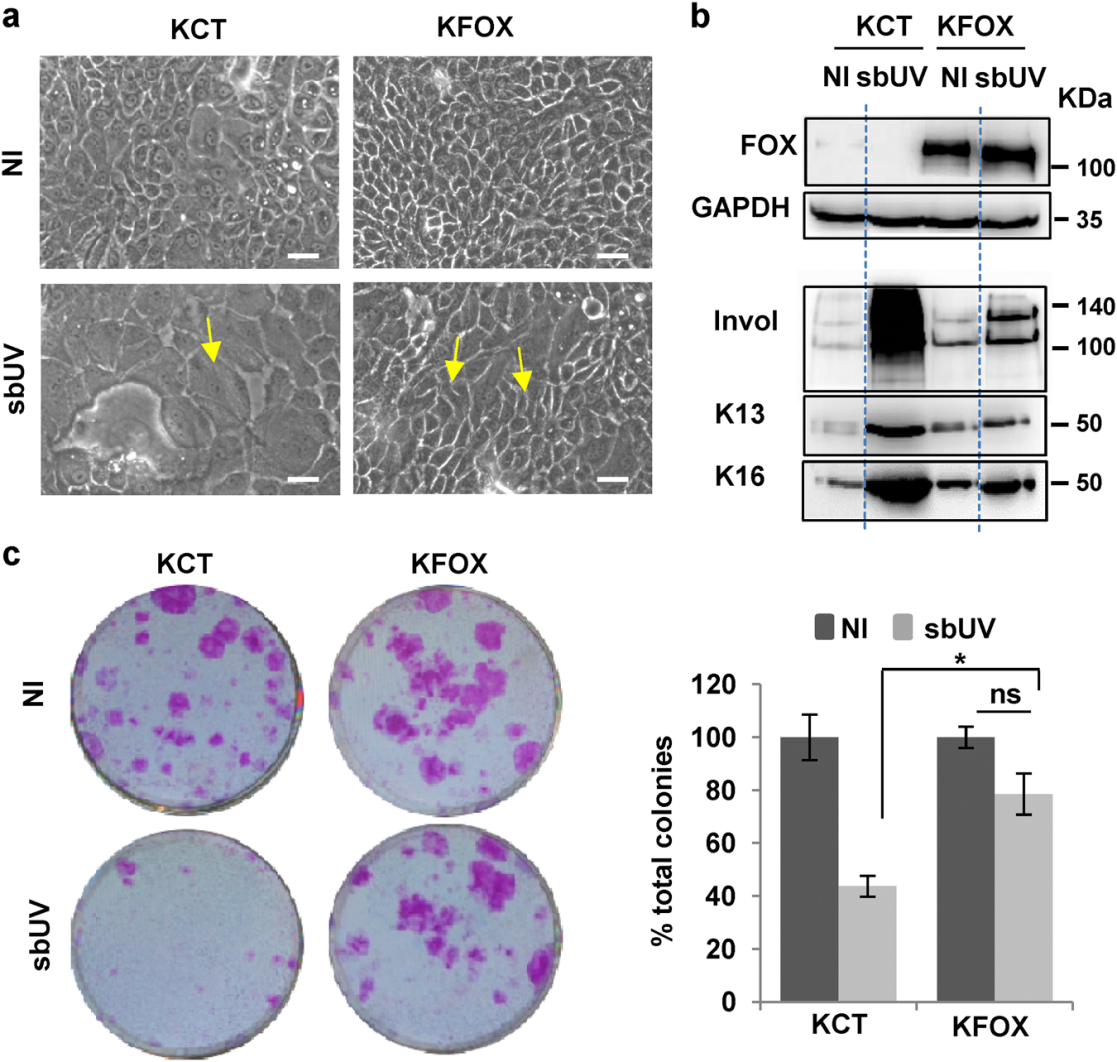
Mitotic regulator FOXM1 suppresses UV-induced squamous differentiation and rescues the clonogenic potential. Analyses of primary human keratinocytes expressing the empty vector (KCT) or the same vector expressing FOXM1 (KFOX), non-irradiated or 48 h after sbUV irradiation. a Representative microphotographs of phase contrast. Note that typical differentiated large cells are induced by UV whereas small proliferative cells are recovered by FOXM1 in spite of UV (yellow arrows). b Western blotting of KCT or KFOX on soluble (FOXM1 and GAPDH as its loading control) or insoluble (Invol, keratins K13 and K16) cellular protein fraction. c Clonogenic capacity of the cells indicated plated 48 h after sbUV irradiation (2500 cells per well in triplicates). Bar histogram shows the percent of colonies relative to CT 48 h after irradiation. NI: non-irradiated.ns: not statistically significant. p* < 0.05. Data are representative or mean ± s.e.m. of triplicate samples. See also Supplementary Figure 7
